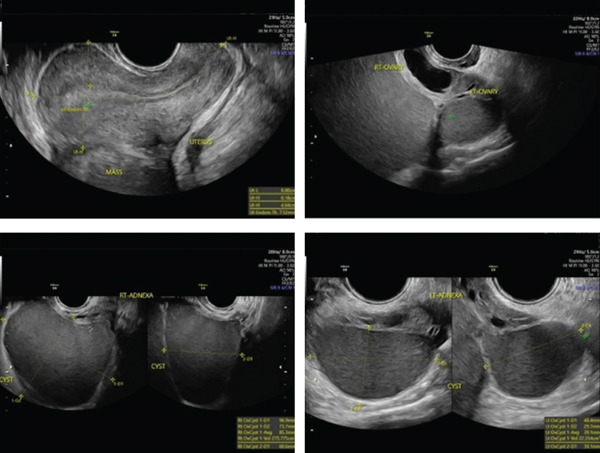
Pelvic ultrasound examination prior to laparoscopic drainage demonstrating bilateral ovarian endometriomas. A large right ovarian endometrioma measuring approximately 10 cm occupied most of the ovary, precluding visualization of antral follicles, whereas the left ovary showed a 5‐cm endometrioma with preserved AFC. Associated pelvic adhesions were also noted.
